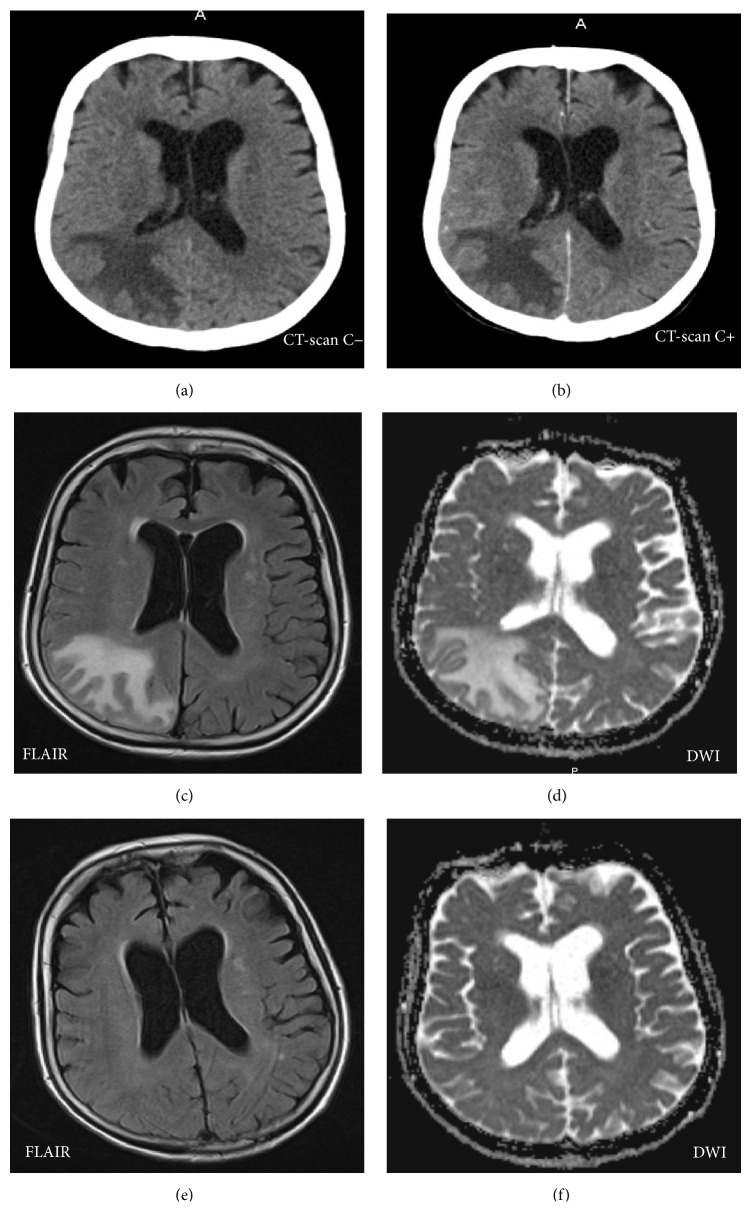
Patient 2: noncontrast brain CT-scan (a) showing a diffuse area of unilateral hypoattenuation in the right white matter in the parietooccipital lobes with a discrete mass effect on the occipital horn of the lateral ventricle, without contrast enhancement (b), in favour of a space occupying lesion of the brain. MRI-scan, axial FLAIR (c) and Diffusion Weighted Imaging, DWI (d), showing an area of FLAIR hyperintensity without diffusion restriction and without contrast enhancement with the same localization as on the CT-scan corresponding to a vasogenic cerebral edema. Follow-up MRI-scan one month later, axial FLAIR (e) and DWI (f), showing the disappearance of the parietooccipital hyperintense area corresponding to a vasogenic cerebral edema, confirming PRES.
